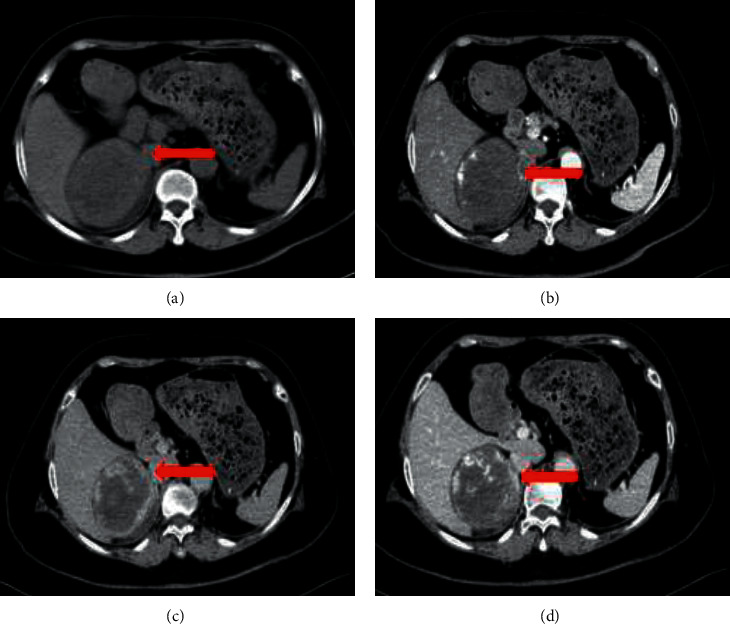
Right adrenal cavernous hemangioma with a tumor size of 8 cm. (a) The unenhanced CT image. (b) Enhanced multiphase CT images revealing early peripheral nodular enhancement in the arterial phase (red arrow). (c), (d) Progressive partial filling-in (red arrow) in the venous phase and delayed phase.
